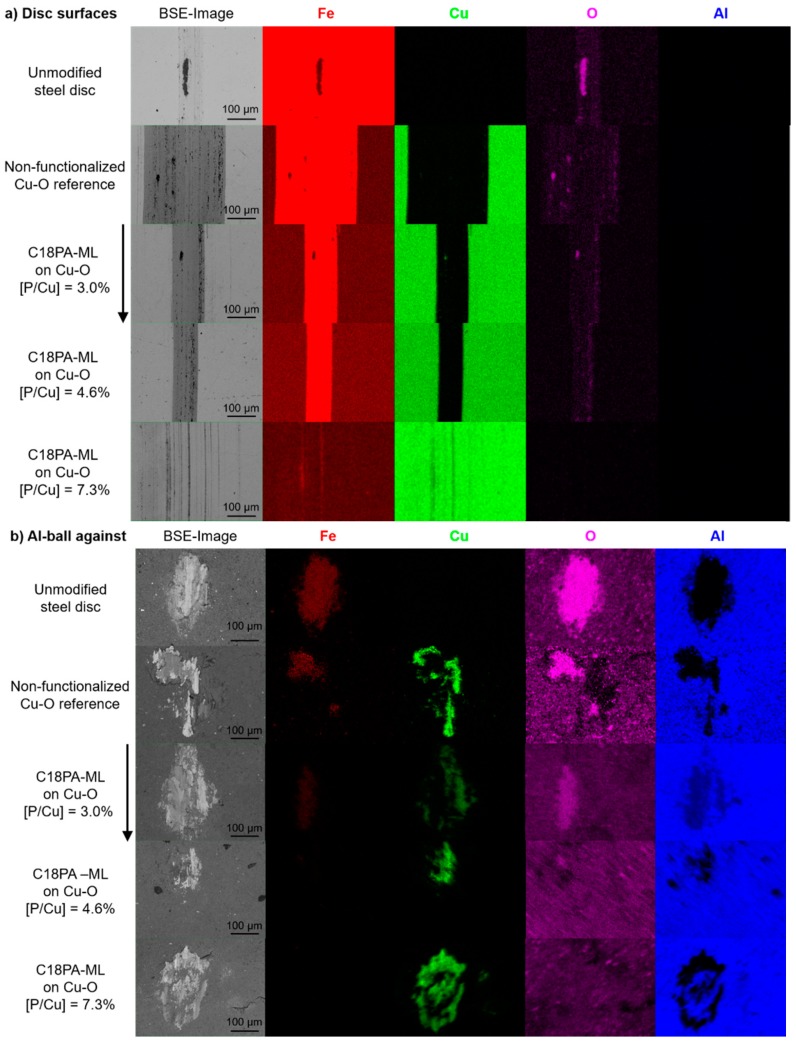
Elemental distribution of Cu–O surfaces coated on tool steel disks without and with C18PA functionalization of different molecular coverages and of an unmodified disk (a) as well as of corresponding aluminum counter-bodies (b) obtained by X-ray analysis (EDX) after ball-on-disk experiments. Coverages are indicated by the P/Cu-ratio, the sliding direction by an arrow.
